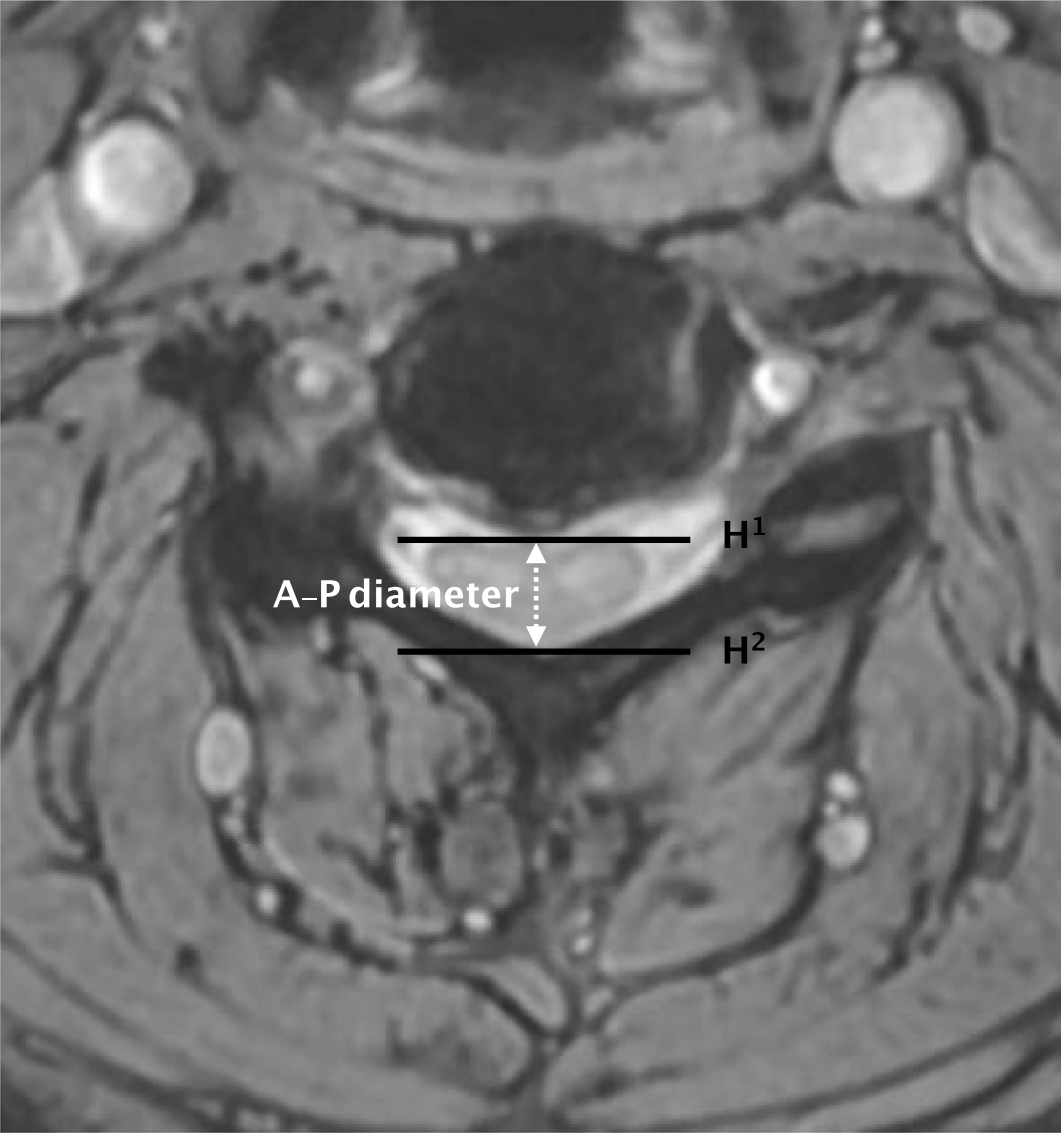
Cross section view of the cervical spinal cord measured on T2-weighted magnetic resonance images. A–P diameter was defined as the highest distance of spinal cord, H1 was the highest and H2 was the lowest points of the spinal canal
